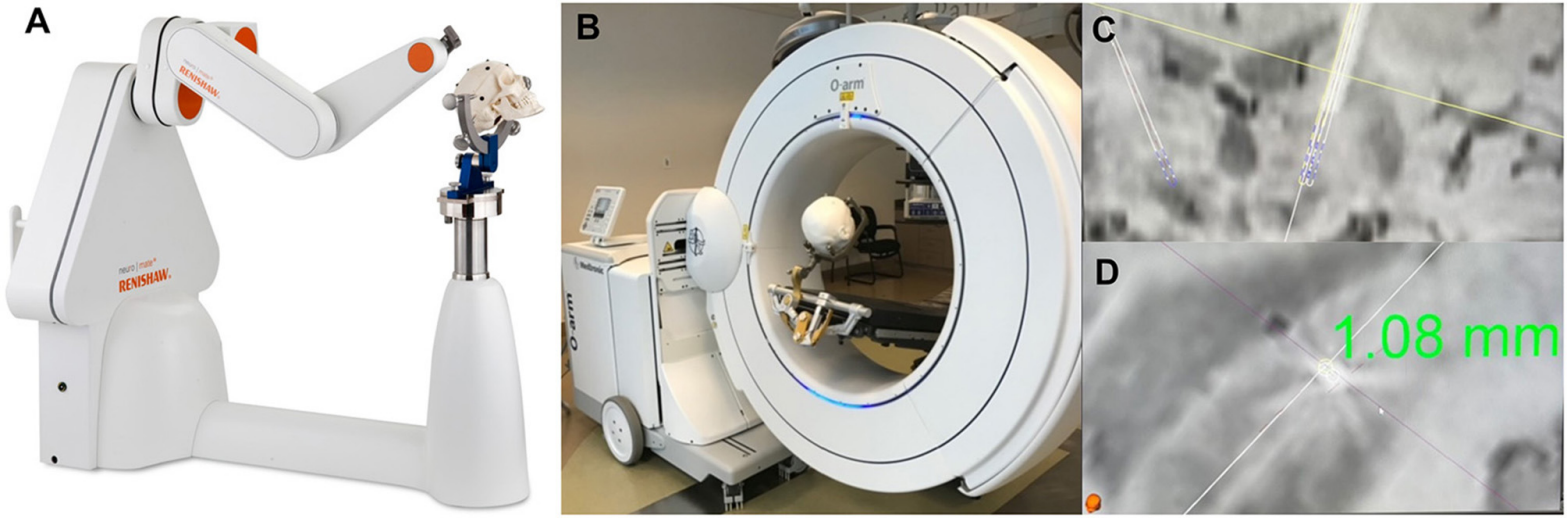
Robotic Surgery assisted by Intraoperative Imaging. The utility of combining intraoperative robotic technology with intraoperative imaging allows for real time evaluation of accuracy of lead placement regarding the preoperative planned trajectory. This allows for adjustment of the cannula trajectory prior to MER recordings or lead placement. (A) Renishaw Neuromate robot. (B) Medtronic O-arm with mock set-up for acquiring intraoperative imaging. (C,D) Intraoperative O-arm image fused with preoperative SWI MRI showing a 1.08 mm lateral deviation from the planned trajectory.
